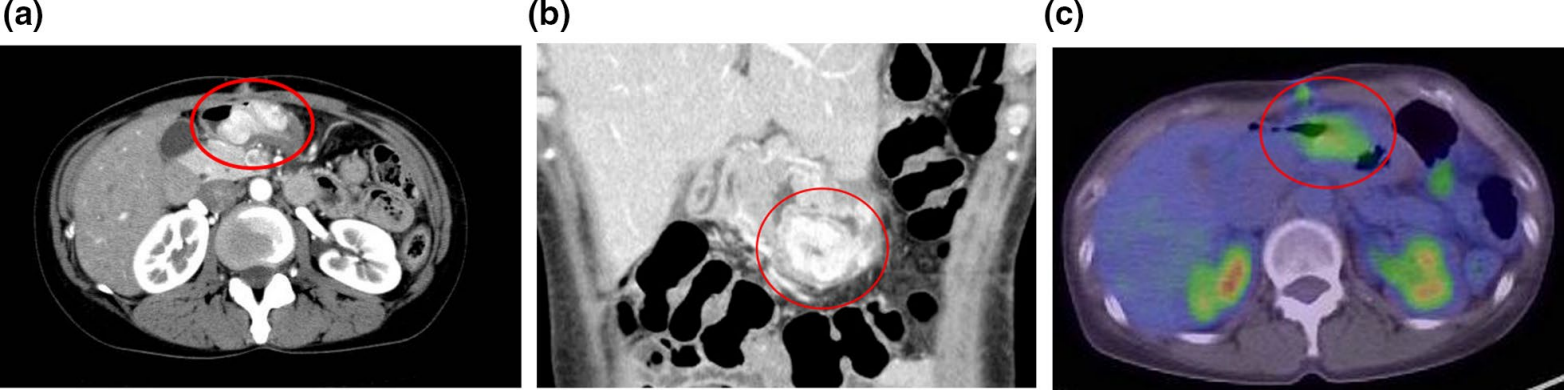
Contrast-enhanced computed tomography (a, b), positron emission, and computed tomography (c). The red line encircles the gastric tumor. a, b The tumor was mainly located in the gastric wall. The fat layer between the stomach and pancreatic head was intact on the dorsal side. c The tumor showed uptake (maximum standardized uptake value: 4.47), whereas no other metastasis was suggested
